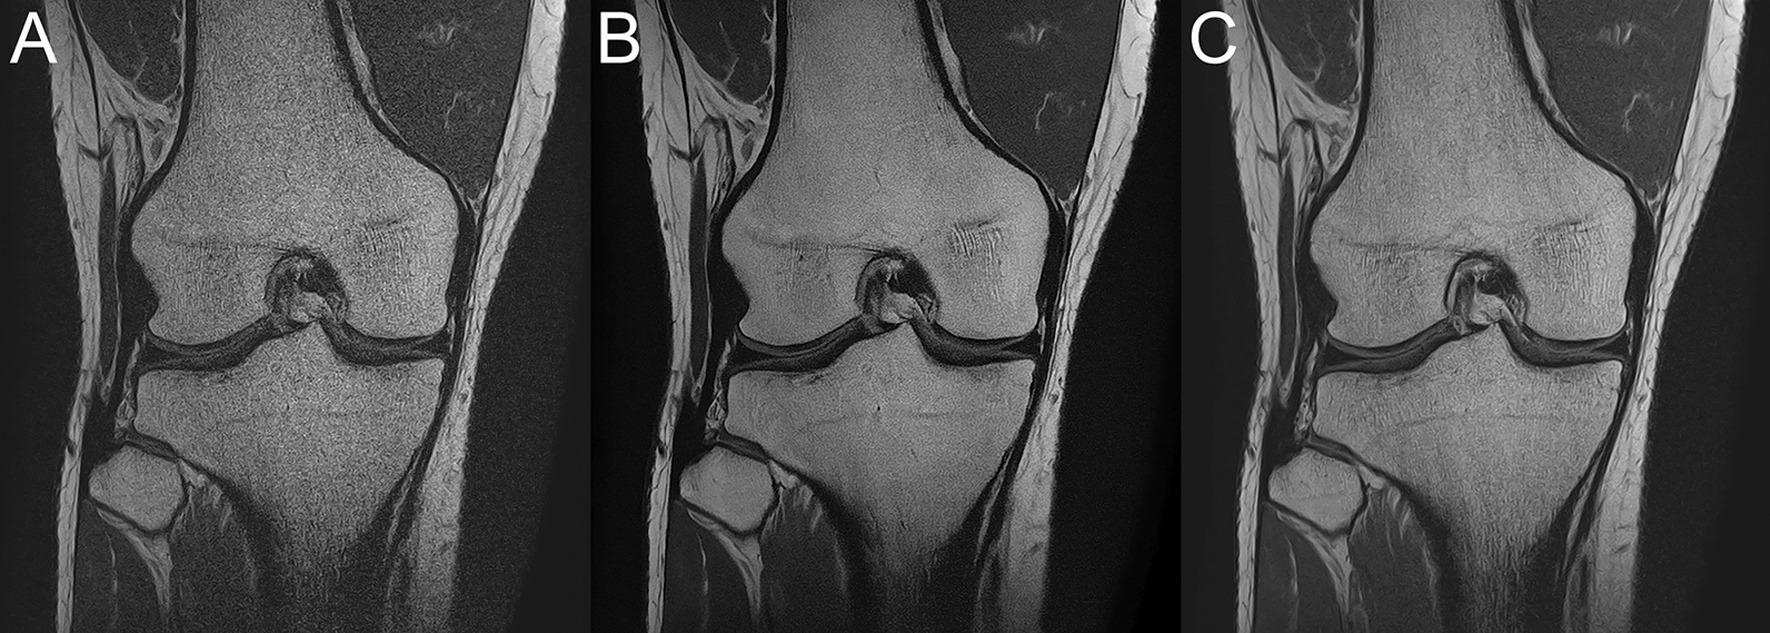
Representative MR images from a 32-year-old healthy male volunteer: A 1NSA image, B 1NSA-DLR image, C 4NSA image. The 1NSA-DLR image exhibits less noise compared with the 4NSA image; the overall diagnostic acceptability scores were 4 for the 1NSA-DLR image and 3 for the 4NSA image (all readers)
